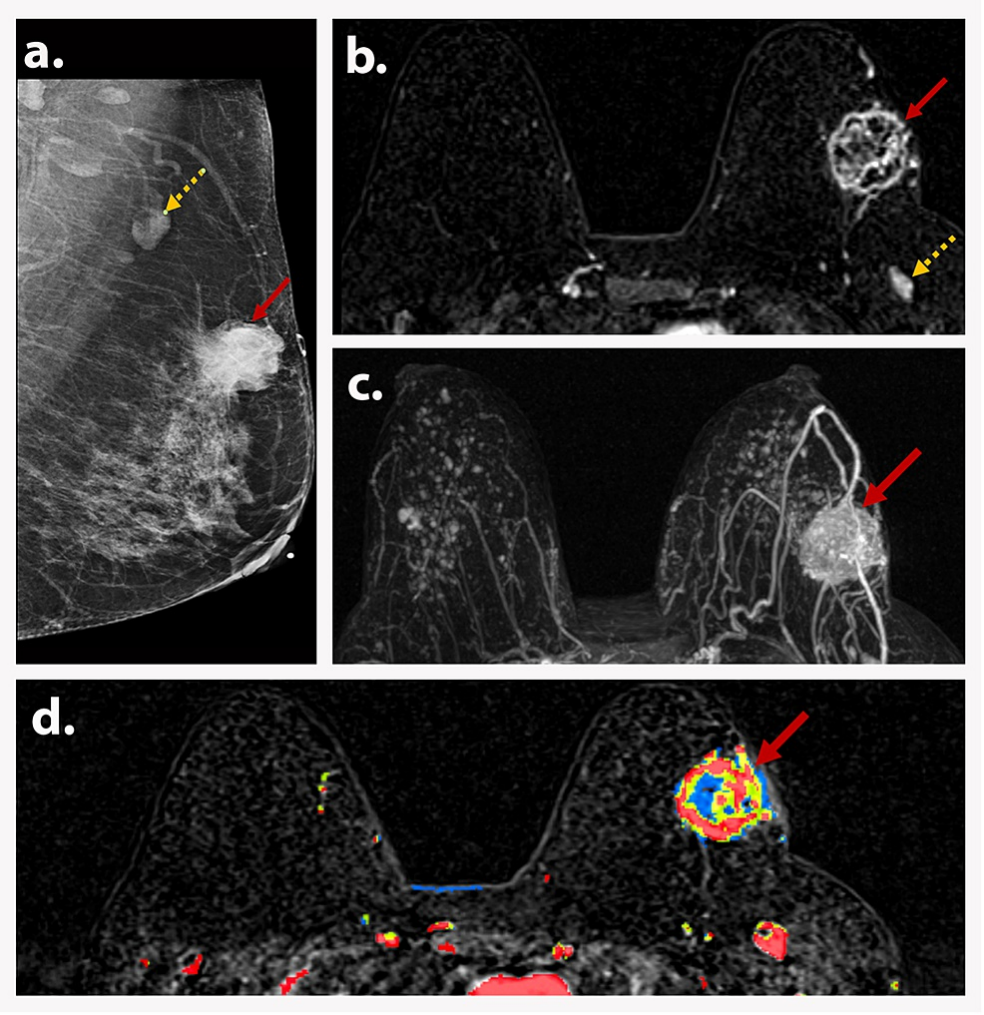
AJCC TNM stage IIB (T2N1M0) A 55-year-old postmenopausal woman presented with a palpable left breast mass. MLO view of the left breast (a) shows a 4 cm oval mass (red arrow) with spiculated and indistinct margins and suspicious axillary lymphadenopathy (dotted yellow arrow). Axial T1-weighted DCE subtraction image (b) shows a 3.9 cm irregular, spiculated, heterogeneously enhancing mass (red arrow) in the left upper outer quadrant with ipsilateral level 1 axillary lymphadenopathy (dotted yellow arrow). Axial MIP (c) and axial T1-weighted DCE with color (d) demonstrate the mass with neovascularization and mixed kinetics (red arrows). Biopsy depicted invasive carcinoma. Findings are consistent with AJCC TNM stage IIB (T2N1M0). AJCC: American Joint Committee on Cancer; TNM: tumor, node, and metastasis; MLO: mediolateral oblique; MIP: maximum intensity projection; DCE: dynamic contrast-enhanced.
